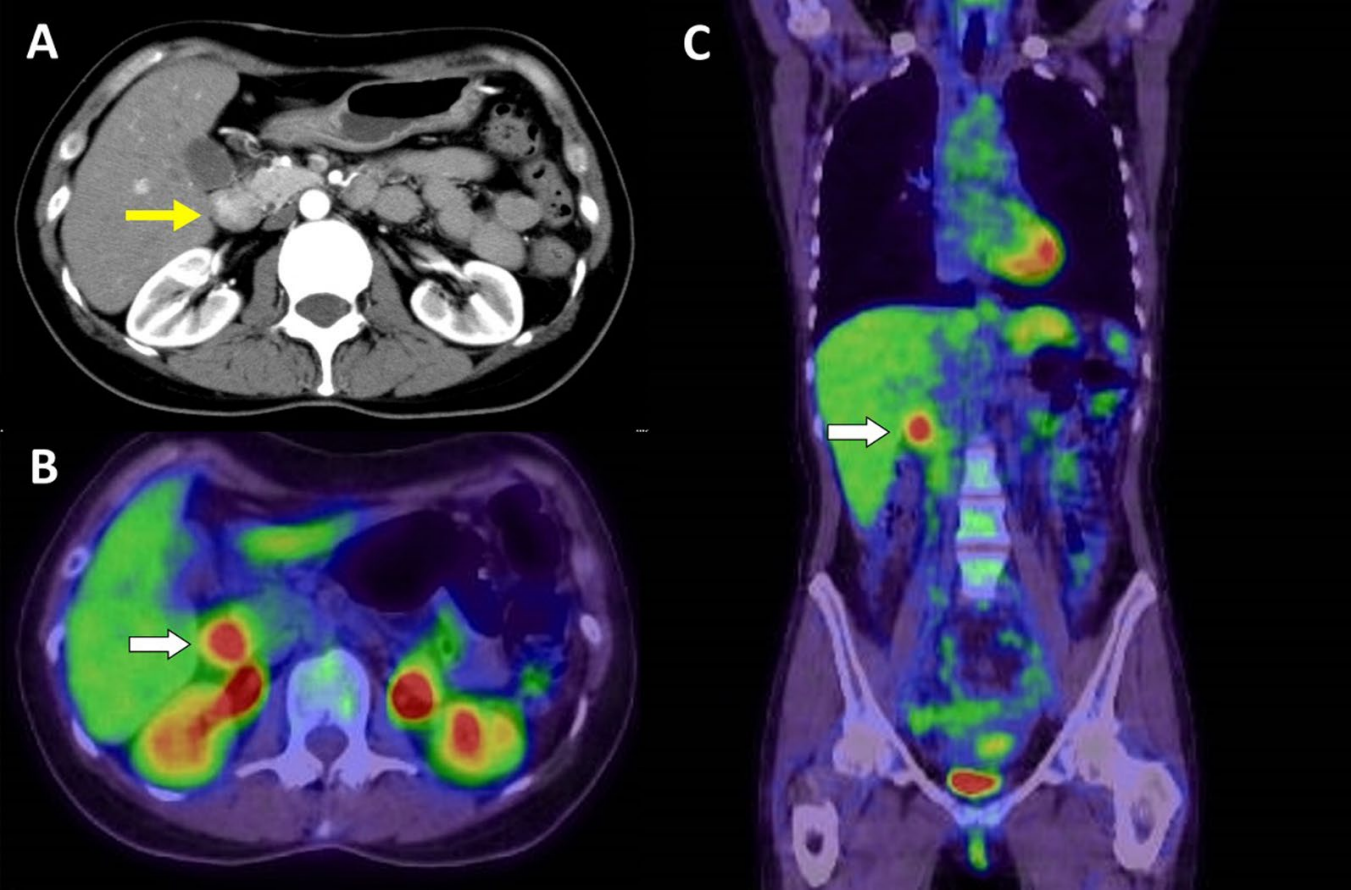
Computed tomography and 18-fluorodeoxyglucose–positron emission tomography images. A Contrast-enhanced computed tomography demonstrated a 20 × 20-mm duodenal mass (yellow arrow). B, C Demonstrated an accumulation of 18-fluorodeoxyglucose in this tumor with a maximum standardized uptake value of 5.78 (white arrows)
